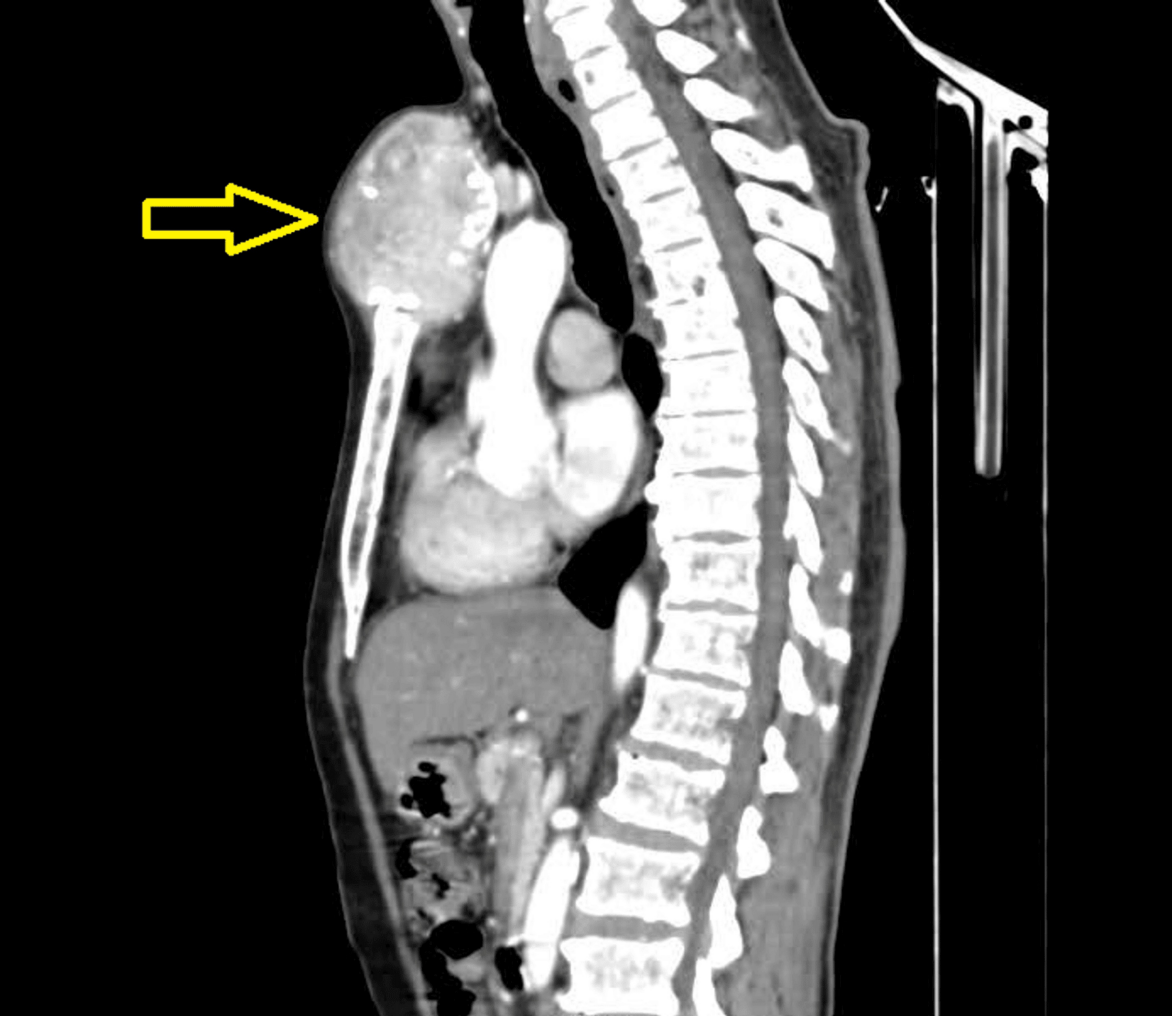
CT scan showing mass lesion (yellow arrow) in manubrium sterni
